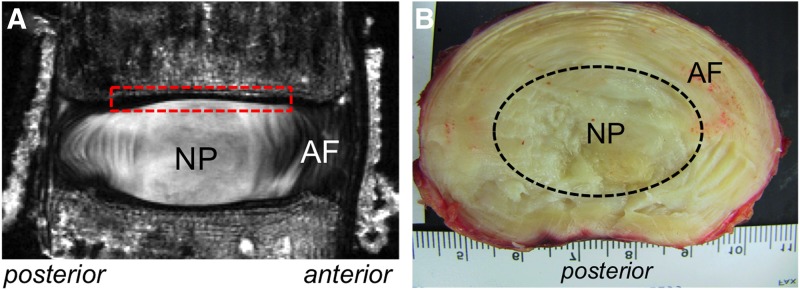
(A) High-resolution magnetic resonance image (MRI) from the midsagittal slice of a nondegenerated lumbar intervertebral disc. Red dashed box represents a region covered by the cartilaginous end plate, which is located on the superior and inferior end of the disc. (B) Cross-sectional view of healthy nondegenerated lumbar disc. The approximated nucleus pulposus (NP) region is outlined by the black dashed oval. Scale in background represents 1 mm increments. The annulus fibrosus (AF) structure can be identified on both images.
